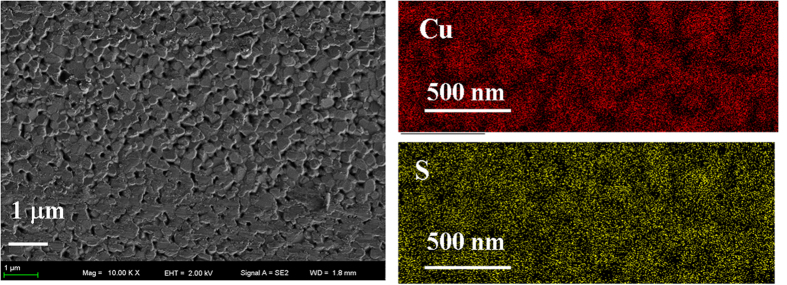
SEM images of the surface morphology (left panel) and x-ray mapping of the elemental distributions (right panel) of the *as-grown* Cu_2_S thin film.
